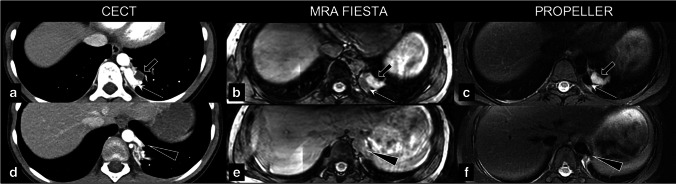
Visualisation of lesion vascularisation on axial contrast-enhanced postnatal CT (a and d), MRA FIESTA (b and e), and T2-w PROPELLER (c and f) in a patient with BPS. Images show venous drainage of the lesion into the hemiazygos vein (thin arrow on a, b, c) and a bronchele (thick arrow on a, b, c) and arterial supply from the aorta descendens (arrow on d, e, f). BPS, bronchopulmonary sequester; CECT, contrast-enhanced computed tomography; MRA FIESTA, magnetic resonance angiography; PROPELLER, periodically overlapping parallel lines with enhanced reconstruction
